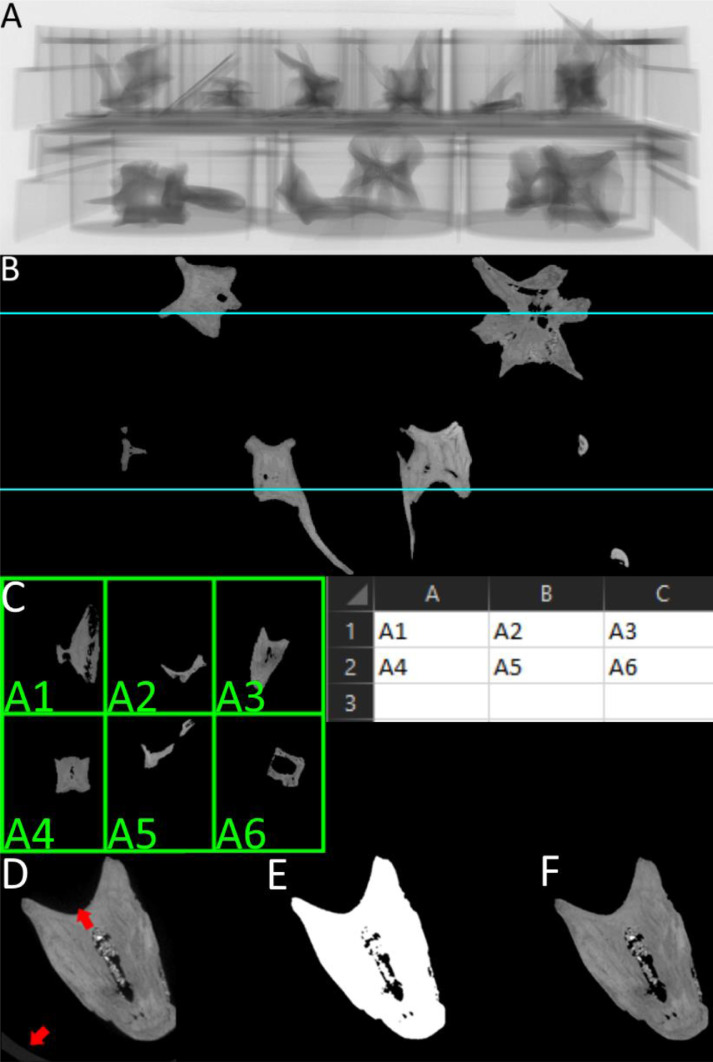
Stages of MiTiSegmenter. A, An radiograph of the stack. B, An example of a thresholded 2 plate stack where the blue line represent the midway point of each tray. C, an example of a labelling grid imported from a CSV file, for visualation the labels are shown in the grid, but in the program only the four corners are labeled. D, a visualisation of raw image, where the microtiter plate and scanner noise is visible. Where the bottom red arrow is the tray, and the top arrow is a edge blur create by the scanner difficult to see in most images. E, an example of a segmentation binary mask, produced that removes the blurred edges and tray. F, The processed image containing only the sample, but preserving internal detail.
